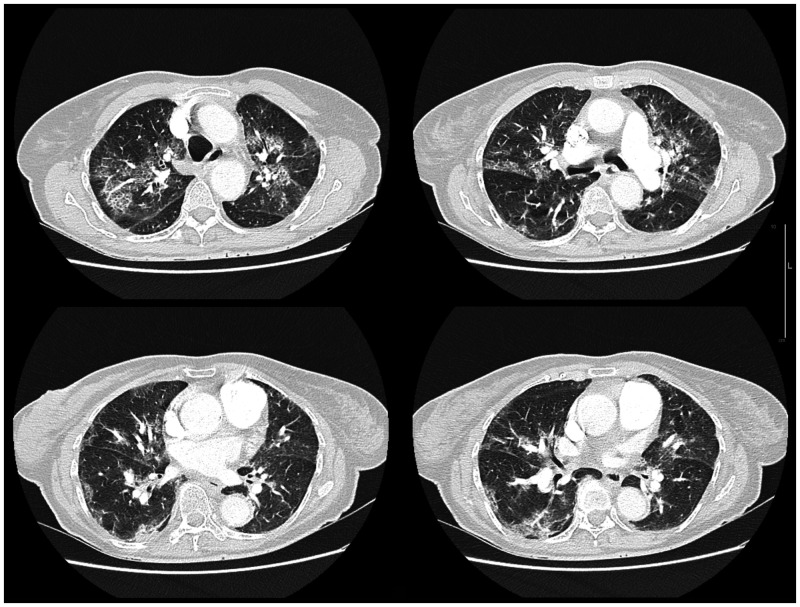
Computed tomographic chest scan at admission, 5 days after symptom onset.
